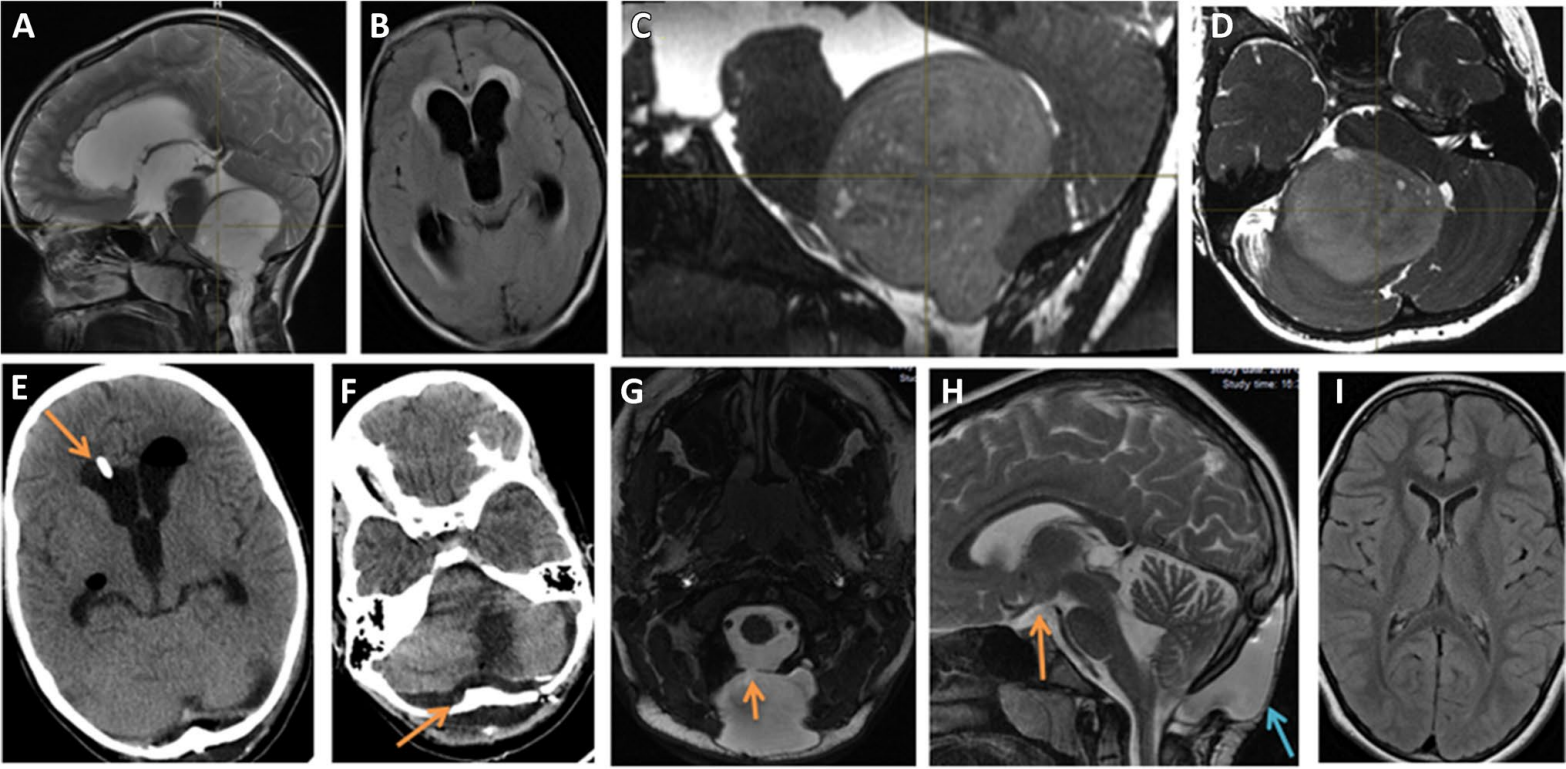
A case of a 7-year-old girl operated for pilocytic astrocytoma of the posterior fossa. (A) Sagittal T2-weighted scan shows a large tumour obliterating the 4th ventricle. (B) FLAIR axial scan demonstrates active HCP. (C), (D) Fiesta 3D sagittal and axial scans show the tumour compressing the brain stem and cerebellum, restricting the CSF flow. (E) Postoperative CT scan performed on day 6 after tumour resection. HCP is still present, although the patient had undergone EVD (orange arrow). For this reason, the patient underwent ETV. (F) CT scan from day 6 shows no evidence of tumour in the posterior fossa. However, there is still cerebellar oedema and some CSF collection in the epidural space (orange arrow). (G), (H), (I) MRI scans performed a year after tumour removal. (G) A large collection of CSF through a dural fistula is shown on the axial Fiesta 3D image (orange arrow). (H) Sagittal T2-weighted image shows the pseudomeningocoele (blue arrow), but, at the same time, good flow of CSF through the area of ventriculostomy (orange arrow) and lack of HCP. A pineal cyst is also present. (I) Axial FLAIR scan shows normal ventricle size after ETV performance
